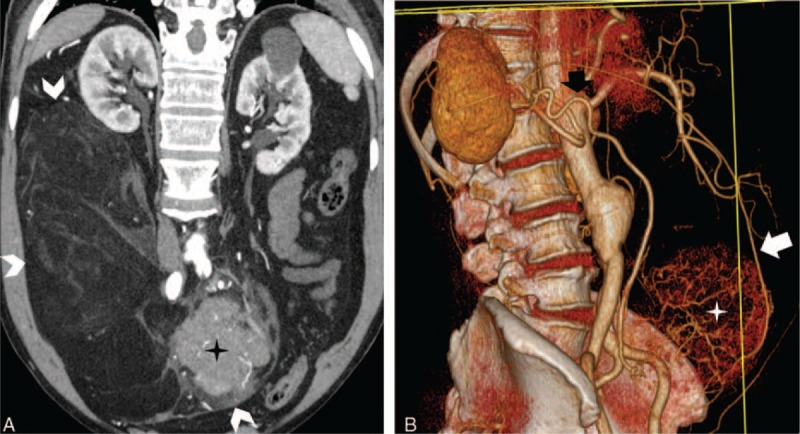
A 64-year-old man with liposarcoma. A, Oblique coronal contrast-enhanced computed tomography image reveals a large fatty tumor arising from right retroperitoneal space (white arrowheads) and a well-defined soft tissue mass within the tumor (cross). B, three-dimensional volume-rendering reconstruction image shows the supply vessel (black and white arrow) of the tumor originating from right adrenal artery and circumventing the kidney.
